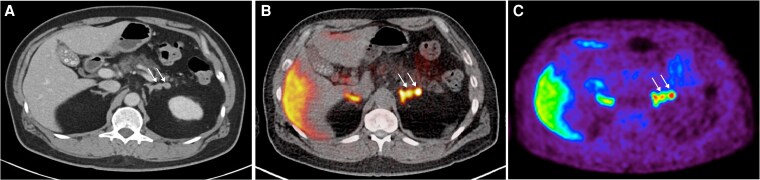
^11^C-MTO PET-CT for case 5—3 small left adrenal nodules detected on CT imaging (A). MTO showed 2 nodules over left lateral limb with high uptake of SUVmax 54.7 (lateral most nodule 1.0 cm) and SUVmax 33.4 (medial most nodule 0.6 cm), compared to the right adrenal gland (34.4) on the fused PET-CT imaging (B) and PET imaging (C). The SUVmax ratio of left adrenal nodules to contralateral gland was 1.59 and 0.97, respectively (compared to cutoff of 1.25 to define lateralization). Abbreviations: ^11^C-MTO PET-CT, 11C-metomidate positron emission tomography-computed tomography; SUVmax, maximum standardized uptake value.
